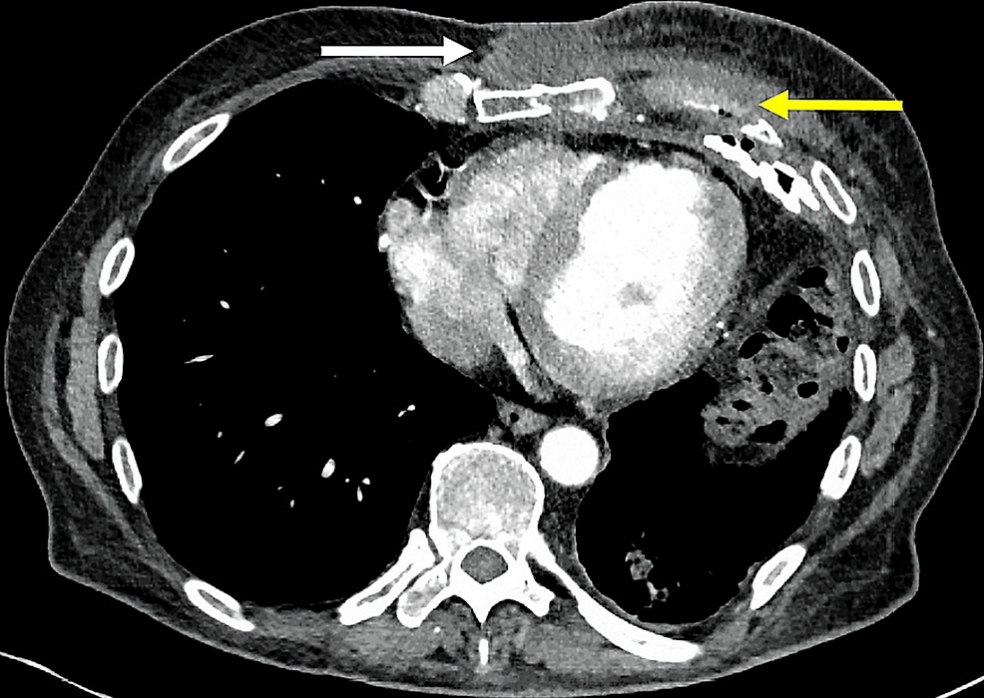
Computed tomography of the chest showing a parasternal fluid collection (white arrow) and osteolysis of the left sixth rib (yellow arrow).
